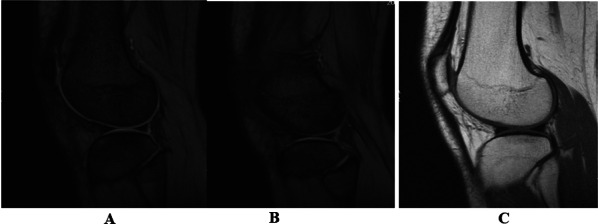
A, B and C MRI post-operative. Successful repair of PMFs both in T1 and T2 in sagittal view
